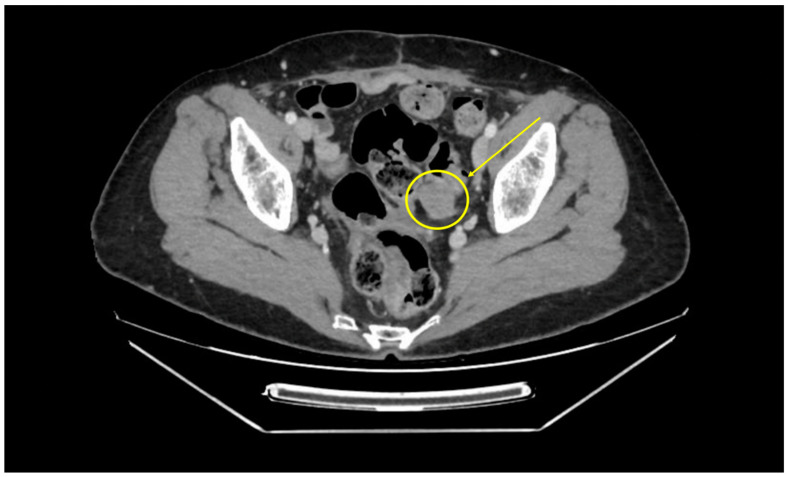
Pre-operative CT-SCAN, analyzed during preoperative multidisciplinary board, showing a rectal lesion of about 3–4 cm adherent to the left parametrium, ureter, and internal iliac vessels at the level of the vaginal stump.
